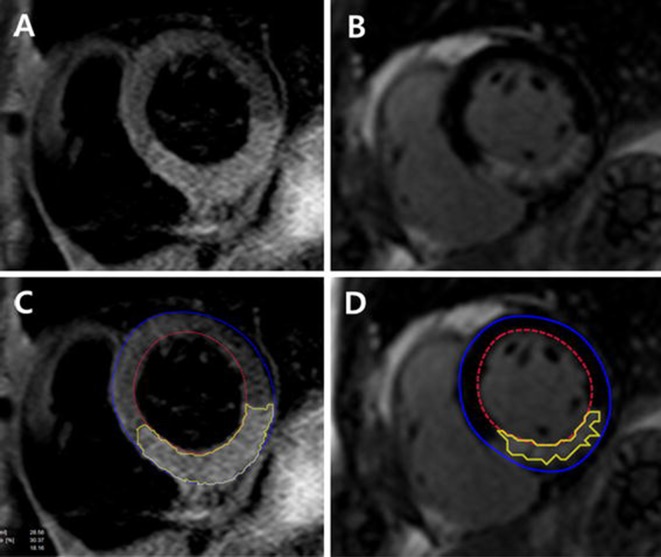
Example CMR images of reperfused inferior STEMI. Short-axis slices of T2-weighted image (A) and the corresponding delayed hyperenhancement image (B) in patients with inferior ST-segment elevation myocardial infarction. The extent of area at risk (C) and infarct size (D) are indicated as by yellow lines.
